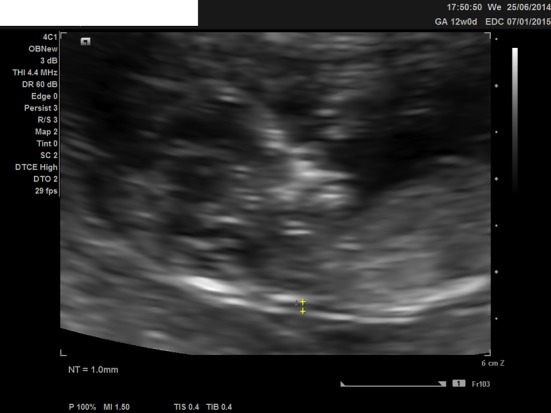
Ultrasound image at 13 weeks, showing the hydrops fetalis.
